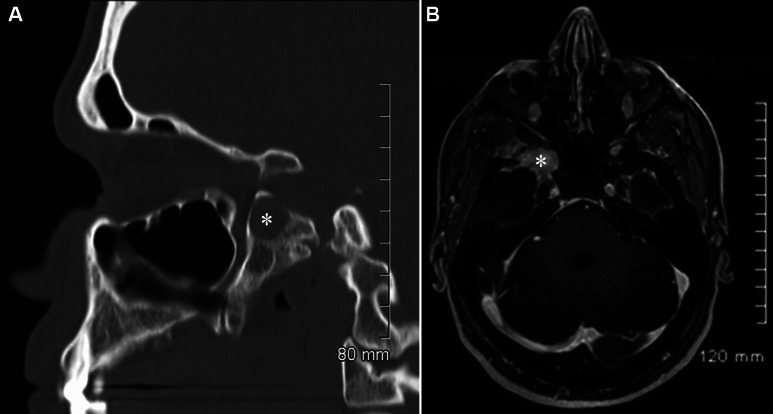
A: Sagittal reformats of the axial non-contrast CT scan revealing the well-demarcated, oval lesion (asterisk) at the sphenoid bone. B: Post-contrast fat saturated axial T1-weight cranial MRI revealing enhancing well-demarcated lesion (asterisk) within the right sphenoid wing around the foramen rotundum.
